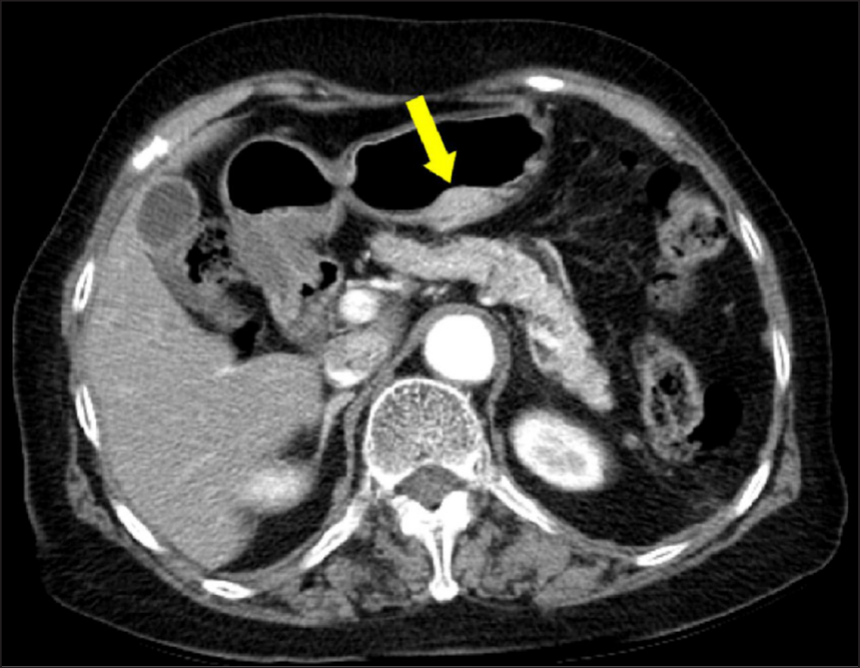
A 79-year-old woman with heterotopic pancreas in stomach. Axial pancreatic phase CT image shows an enhancing mass (arrow) in gastric antrum. The mass reveals ill-defined border and endophytic growth.
